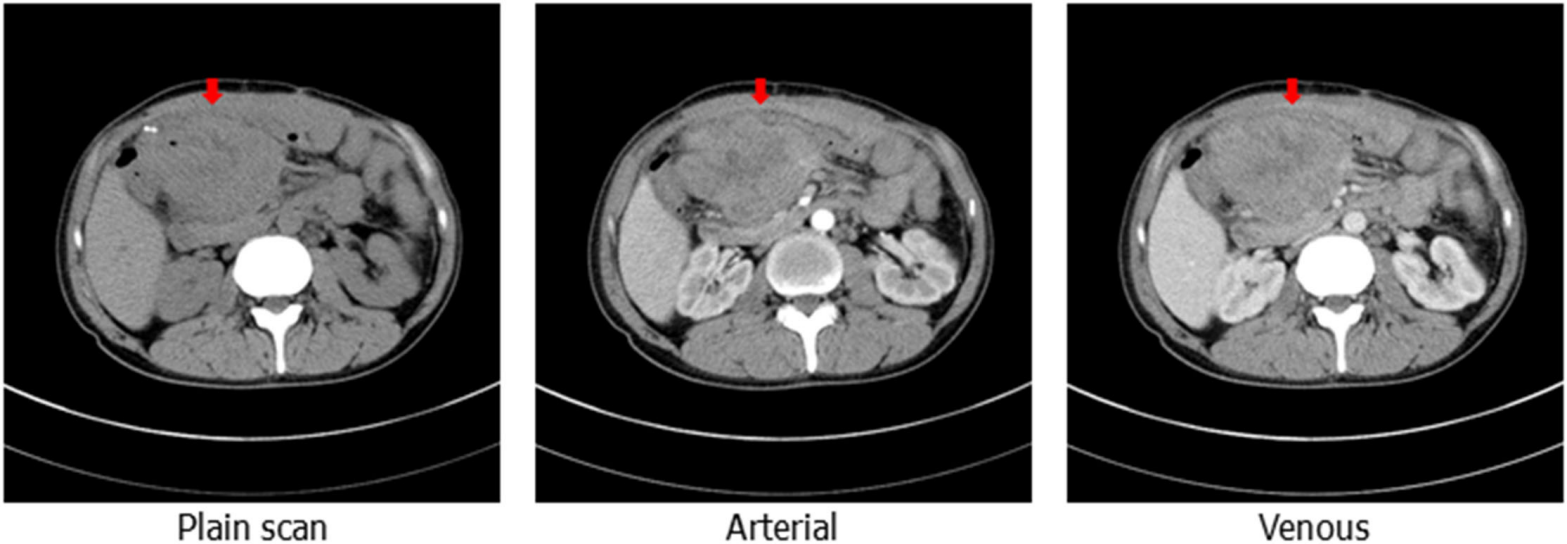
Computed tomography of the abdomen showing the upper abdominal mass with an unclear boundary with the surrounding intestinal canal, obvious enhancement can be observed on enhanced scanning (the red arrow shows the location of the package).
